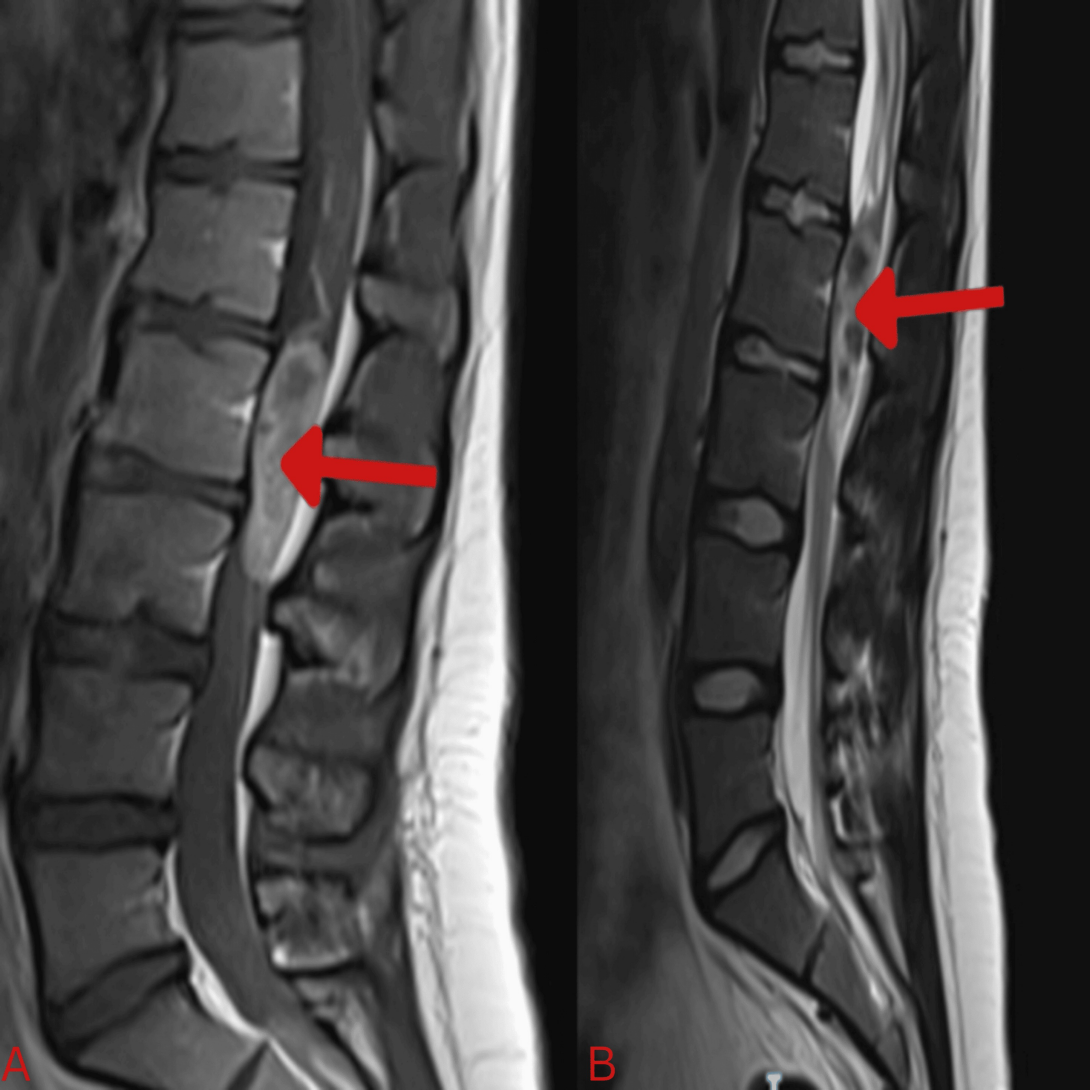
Sagittal T1(A) and T2(B) with contrast MRI of lumbo-sacral spine revealing the lesion, its radiological characteristics and extent. MRI, magnetic resonance imaging
